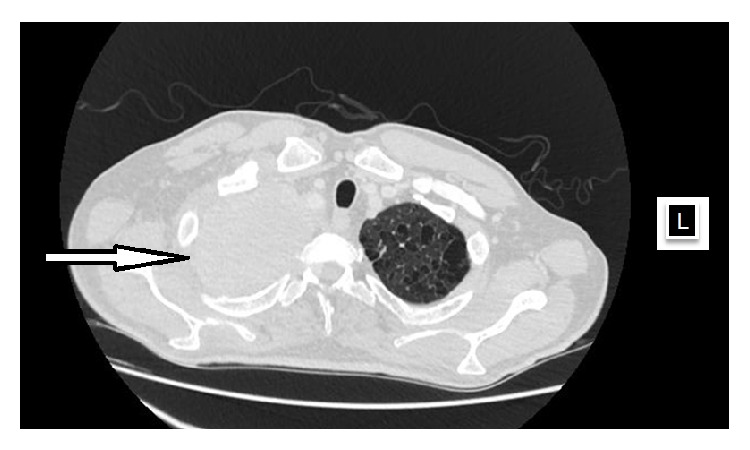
Thorax CT image showing situs inversus and an 11 cm mass opacity at the apex of right lung.
